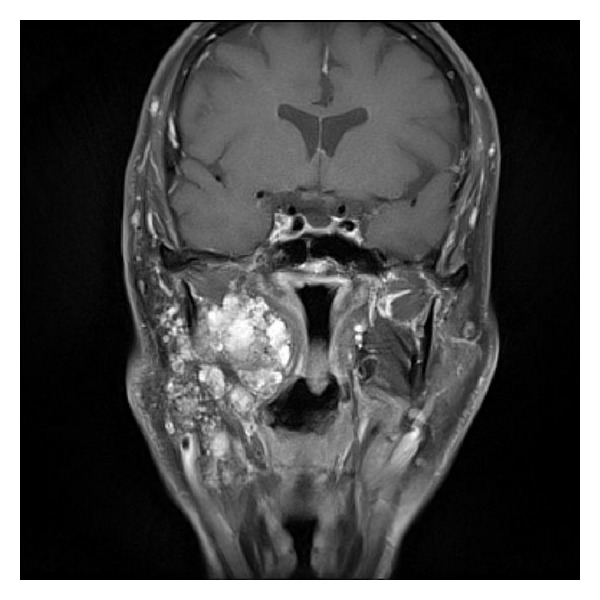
Preoperative MRI T1 Fat Sat gadolinium.
